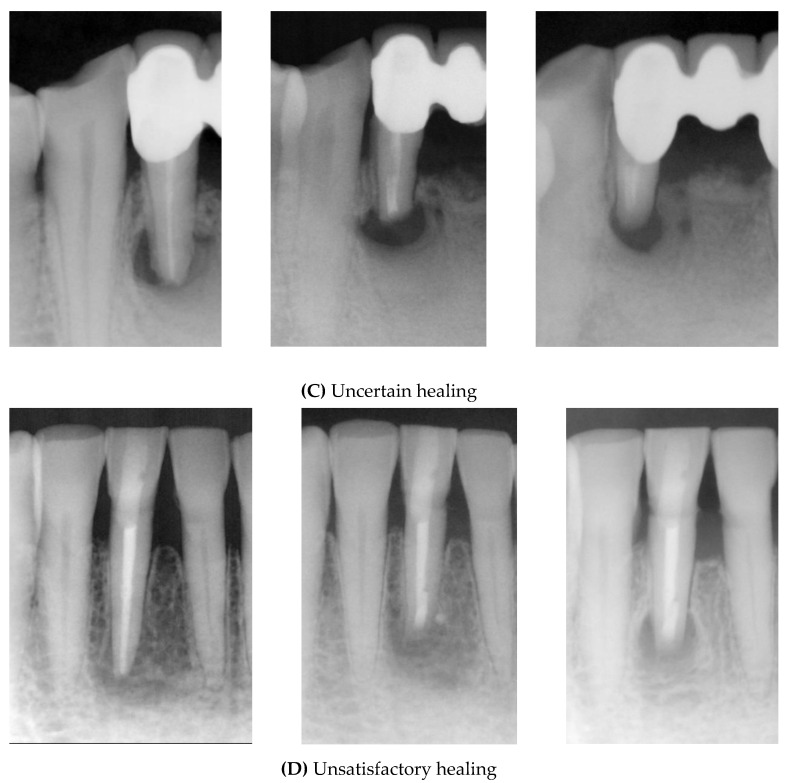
Periapical radiographs demonstrating healing outcomes. (A) Complete healing; preoperative (left), postoperative (center), and 14-year follow-up (right) radiographs showing complete healing of #15 and #16. (B) Incomplete healing; preoperative (left), postoperative (center), and 14-year follow-up (right) radiographs showing incomplete healing (scar tissue) of #12. (C) Uncertain healing; preoperative (left), postoperative (center), and 8-year follow-up (right) radiographs showing uncertain healing of #42. (D) Unsatisfactory healing; preoperative (left), postoperative (center), and 4-year follow-up (right) radiographs showing unsatisfactory healing of #41.
